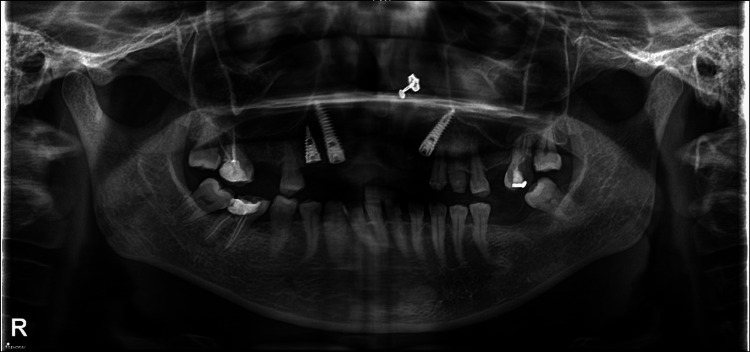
Post-operative orthopantomograph (Case 1)
